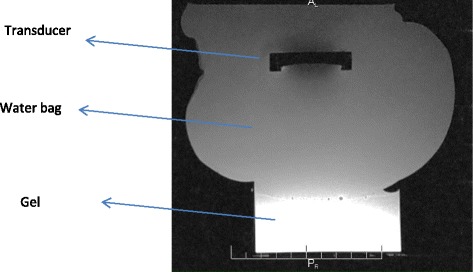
MR image using T2-W FSE of the coupling to the gel of the robotic system
